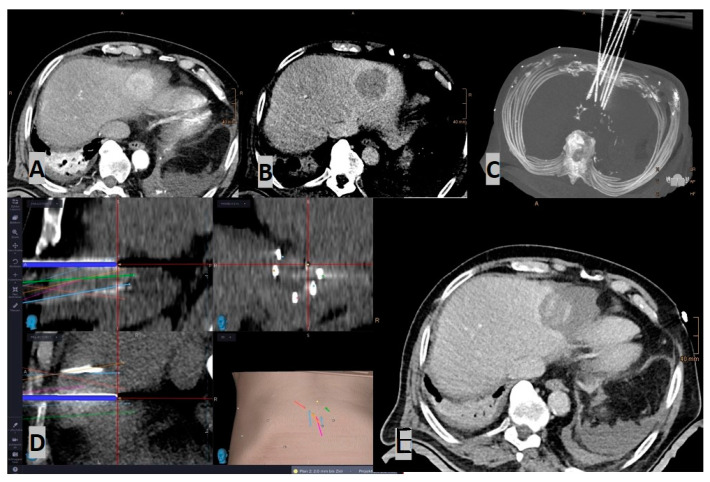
Case of a stereotactic radiofrequency ablation (SRFA) in an 85-year-old male with a sub-cardiac HCC (4.8 cm): (A) Arterial phase planning CT; (B) Portal-venous phase planning CT; (C) MIP of the control CT, showing in total 7 inserted coaxial needles; (D) Screenshot of the frameless stereotactic navigation system: Superposition of the needle control CT on the planning CT, with the pathways showing precise placement of all needles; (E) Contrast-enhanced control CT (portalvenous phase), showing the large ablation zone covering the HCC, including a sufficient safety margin, which was confirmed by image fusion.
